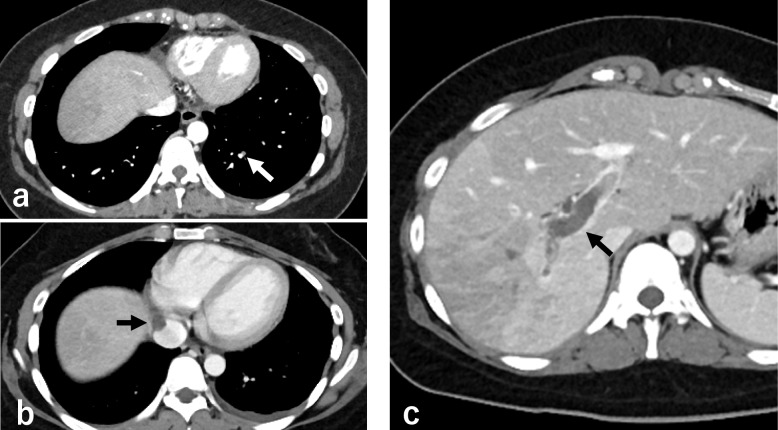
Abdominal contrast CT image findings on day 20. a Pulmonary embolism. b Inferior vena cava thrombosis. c Portal vein thrombosis
